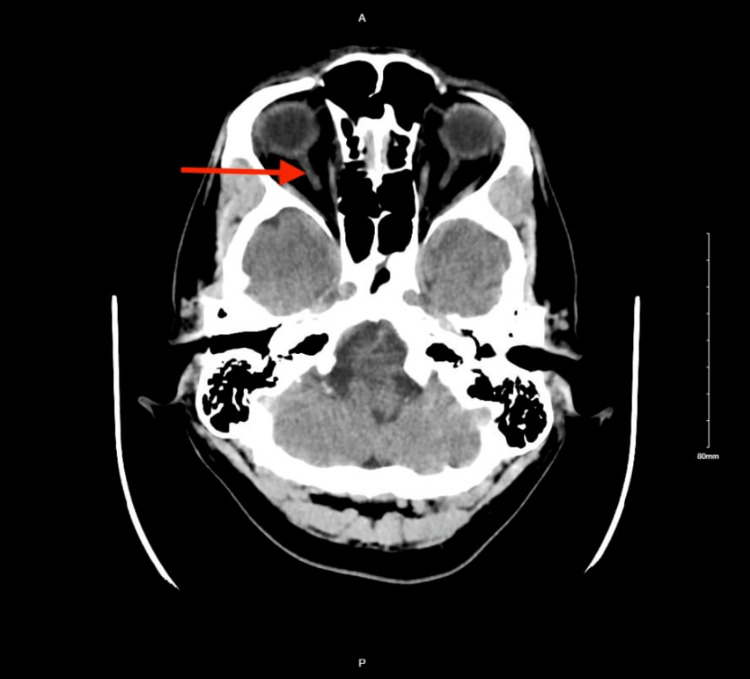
Cerebral CT with the red arrow showing the normal optic nerve
